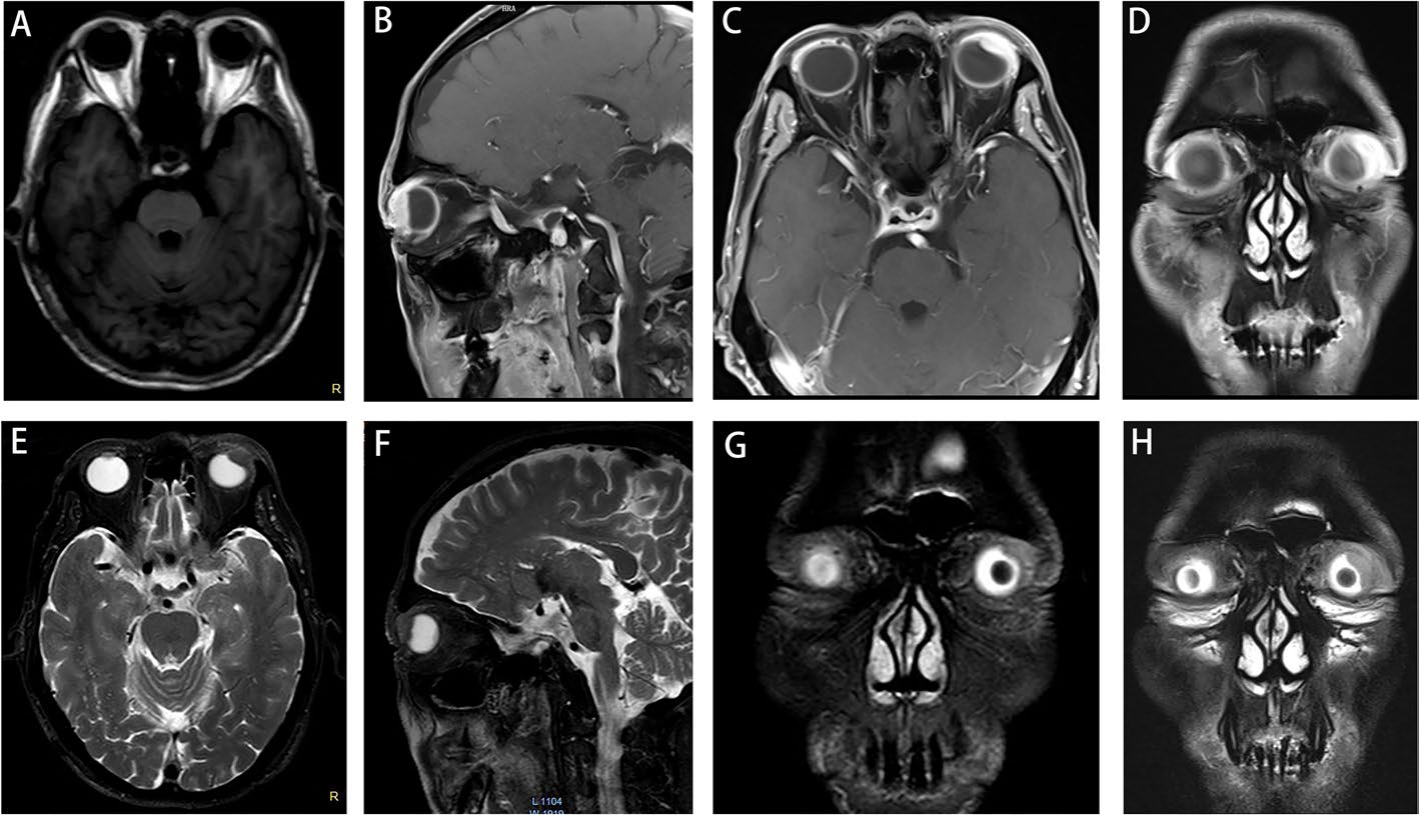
Magnetic resonance imaging (MRI) images of the left orbit at the patient’s first visit before enucleation of the left eyeball. A The lesion located in the ciliary body region of the left eye appeared isointense or slightly hyperintense on T1-weighted image (T1WI). B-D In T1WI post gadolinium enhancement MRI, the contrast enhanced scan showed enhancement in the lesion. The enhanced images manifested hyperintense on T1-f12d fat suppression (fs) (B: T1-f12d-fs-sag; C: T1-f12d-fs-tra; D: T1-f12d-fs-cor). E-H The lesion located in the ciliary body region of the left eye appeared hypointense on T2-weighted image (T2WI) (E: T2WI-tra; F:T2WI-sag; G: T2WI-cor). H The images manifested hypointense and more clear on T2-tse-fs (H)
